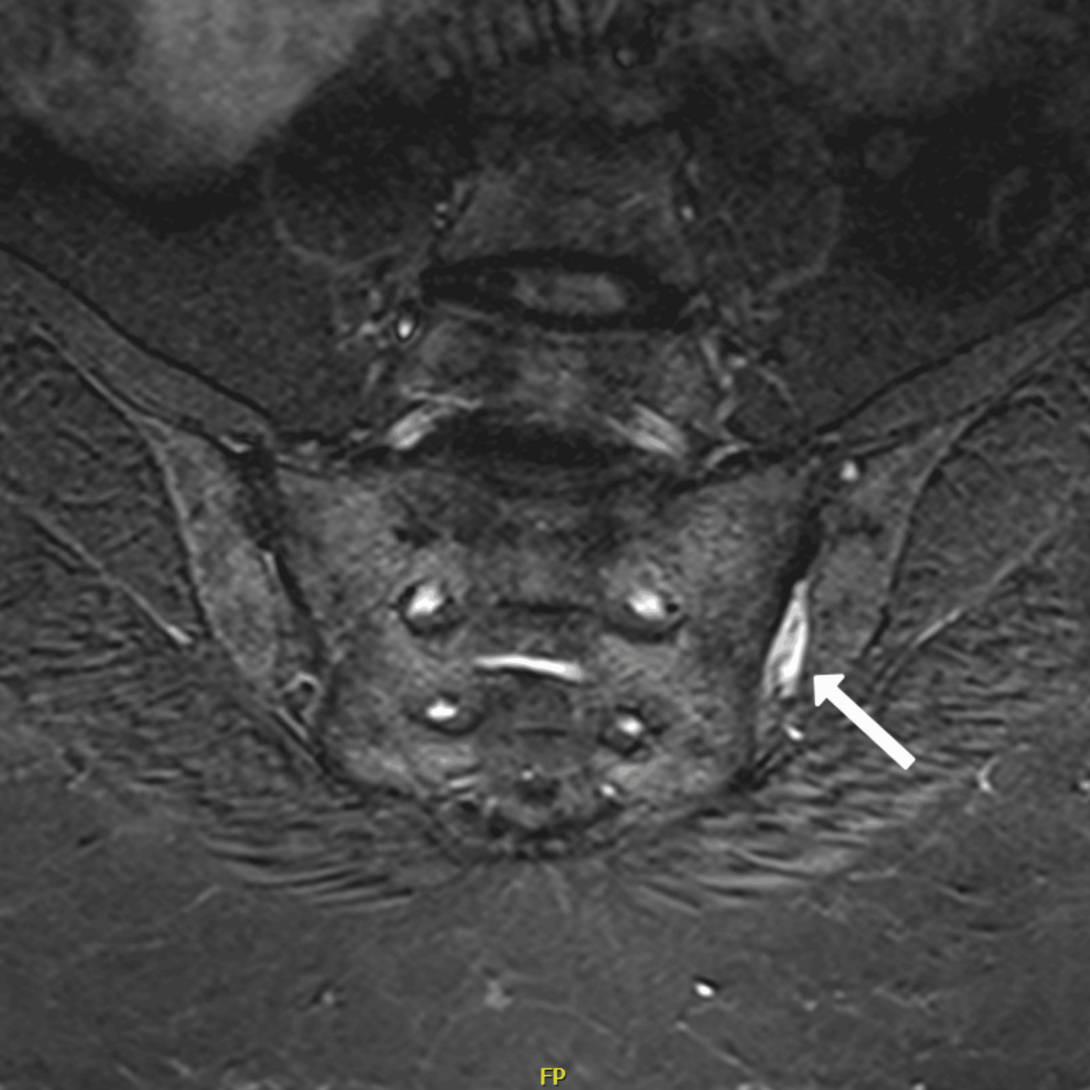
STIR-sequence MRI of the sacro-iliac joints showing left sided inflammatory changes (white arrow) STIR: Short tau inversion recovery
